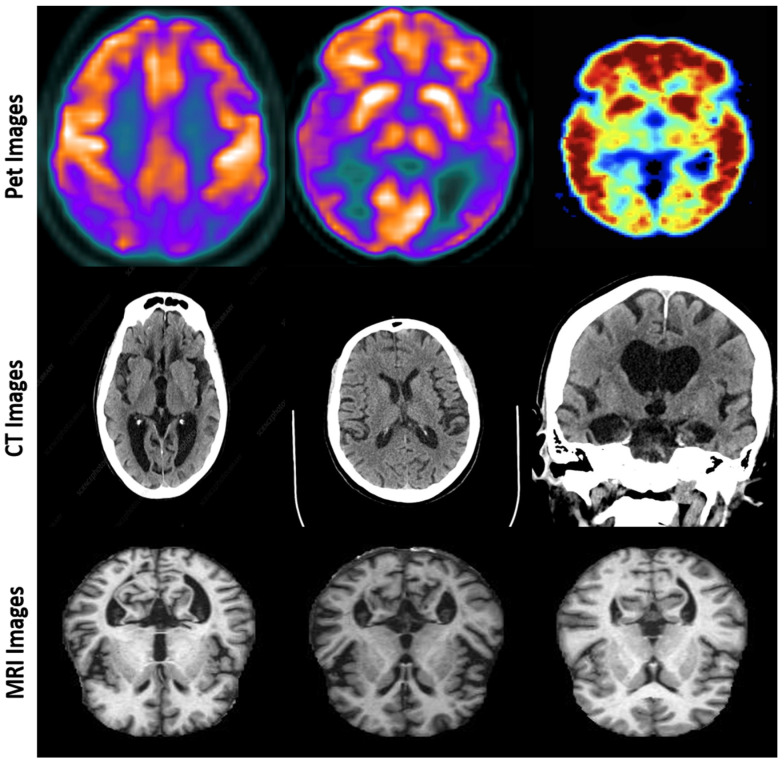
Representative multi-modal imaging data: PET, CT, and MRI scans for Alzheimer’s disease diagnosis.
